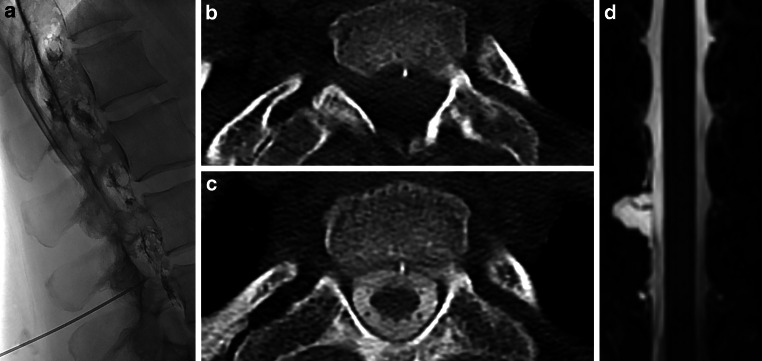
a Distribution of blood and contrast agent in the epidural space during epidural blood patch. b Discogenic microspur on an axial CT image. c Same level after contrast agent injection. d Coronal T2-weighted MRI showing a ruptured nerve root cyst and adjacent epidural CSF collection
